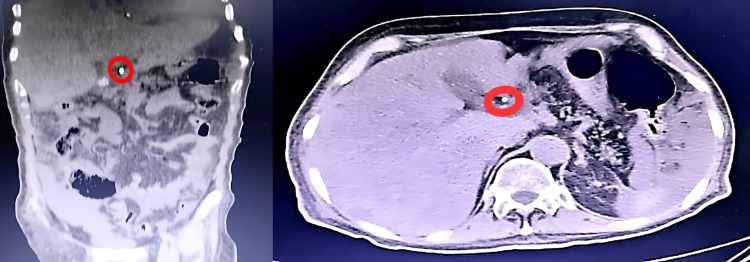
High-resolution axial and coronal contrast-enhanced CT images of the abdomen in a 62-year-old man with jaundice. (a) Coronal CT image shows a hyperdense (calcified) focus within the distal common bile duct (red circle). (b) Axial CT image confirms the hyperdense intraluminal focus, consistent with choledocholithiasis. Note the mild dilation of the upstream biliary duct CT: computed tomography
